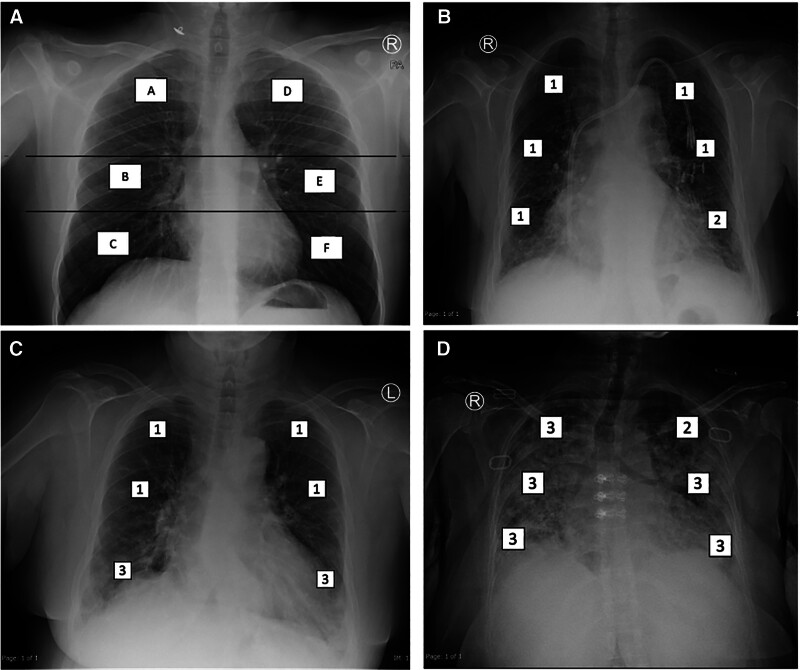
(A) Frontal chest X-ray demonstrating the levels (horizontal lines) and zonal anatomy (A–F) of the Brixia score; (B) frontal chest X-ray for a 77-year-old female at admission due to H1N1, demonstrating the Brixia score with interstitial predominance; (C) frontal chest X-ray in COVID-19 male patient: predominantly interstitial markings in lungs; (D) frontal chest X-ray in COVID-19 male patient: predominantly interstitial markings in lungs at admission; (E) frontal chest X-ray in COVID-19 male patient: predominantly alveolar infiltrates bilaterally; (F) frontal chest X-ray in H1N1 female patient: predominantly alveolar.
